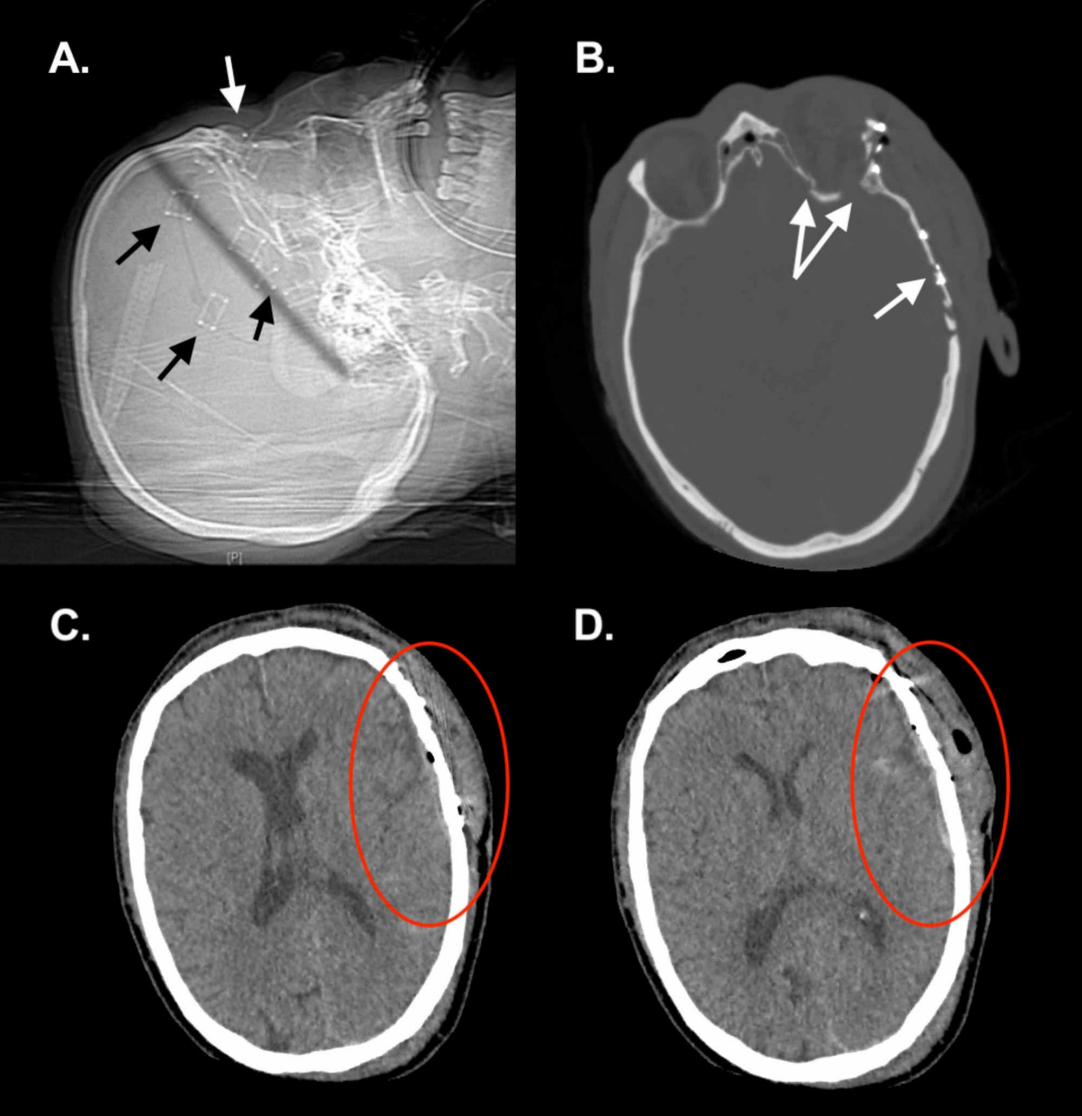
Postoperative radiography at 18 hours (non-contrast CT) and 18 days (MRI). (A) Sagittal scout CT with placement of temporal (black arrows) and orbital (white arrow) miniplates. (B) Reduction of comminuted temporal and lateral orbital wall fractures (bone window). A medial and posterior orbit fracture is still present. (C) Cleared subarachnoid hemorrhage (red oval; brain window) with minimal pneumocephalus appreciated. (D) Evacuated epidural hematoma (red oval; brain window).
